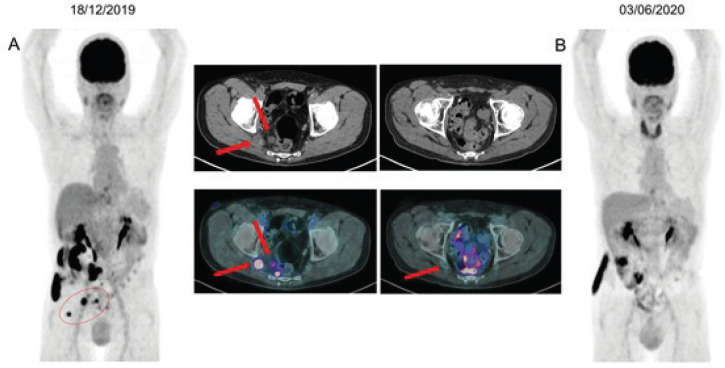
(a): 18F-FDG PET/CT imaging baseline before the initial therapy with pembrolizumab, showing mesorectal and inferior mesenteric LN, measuring up to 1.7 cm (SUVmax: 7.9). Nucleus centred on the right sciatic foramen measuring 2.0 cm (SUVmax: 10.4), maintaining close contact with the sciatic roots on this side. (b): Restaging after seventh cycle demonstrating resolution of glycolytic hypermetabolism in those previous LN.
